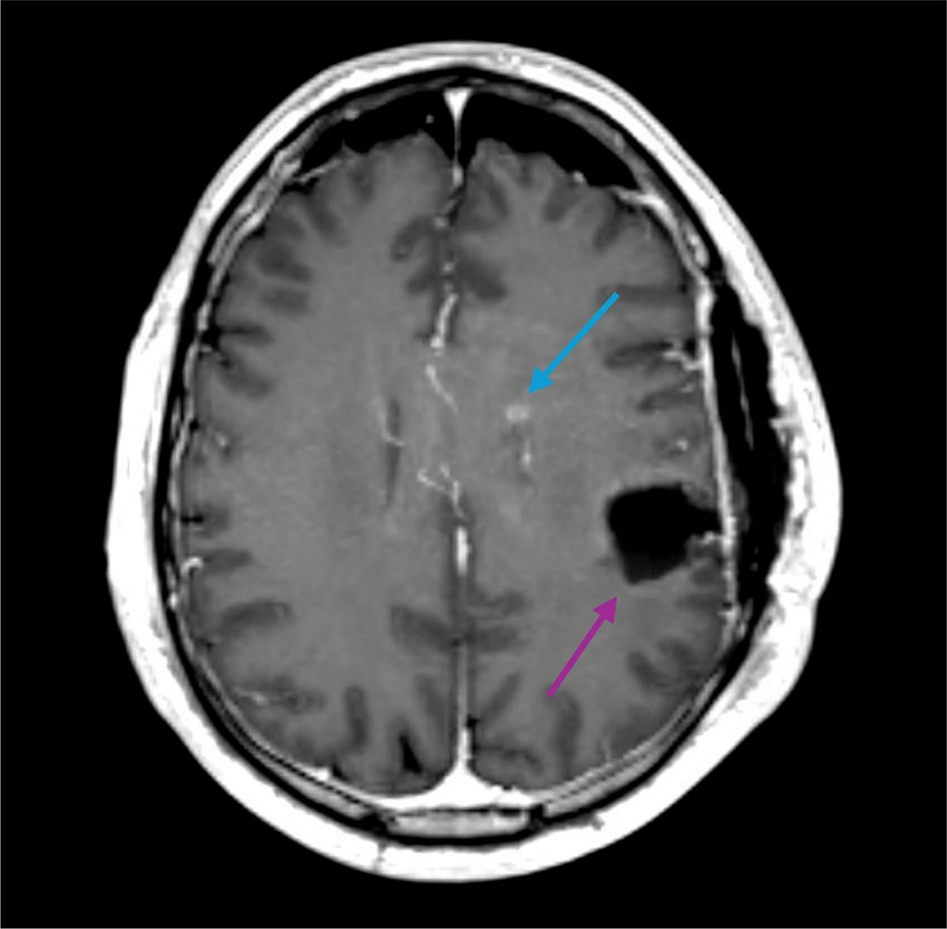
Axial gadolinium-enhanced T1-weighted image of a patient with a distant tumour (yellow arrow). The distant tumour is seen anteromesial to the resection cavity (blue arrow).
